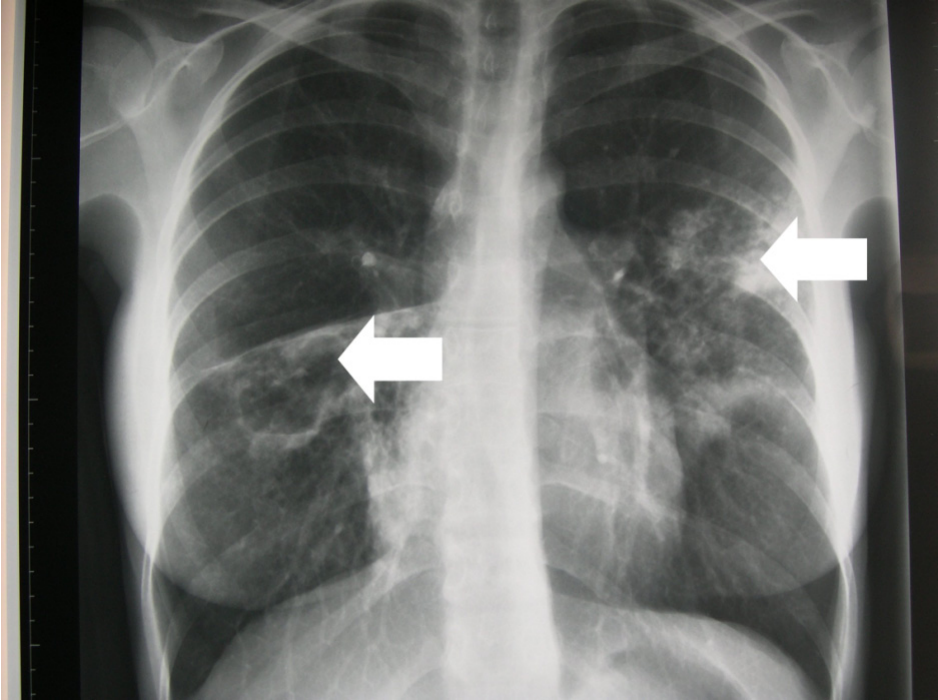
Chest radiograph demonstrating an area of infiltration in the left upper lobe and a cavitating lesion in the right middle lobe.
